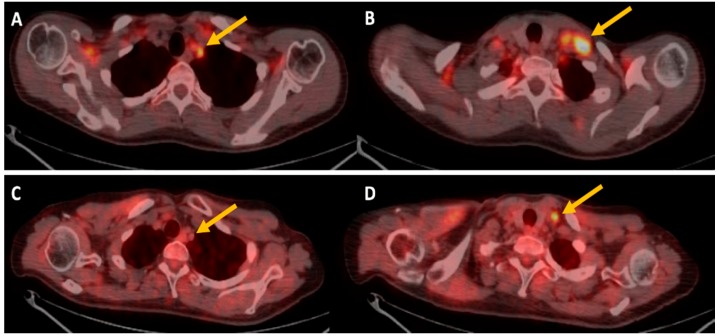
^18^F-FDG PET/CT imaging of a patient treated with bevacizuamb. (A,B) are transaxial PET/CT images at baseline that shows high ^18^F-FDG uptake in left supraclavicular lymph nodes (yellow arrows), (C,D) transaxial PET/CT images after therapy shows reduction of ^18^F-FDG uptake (yellow arrows).
